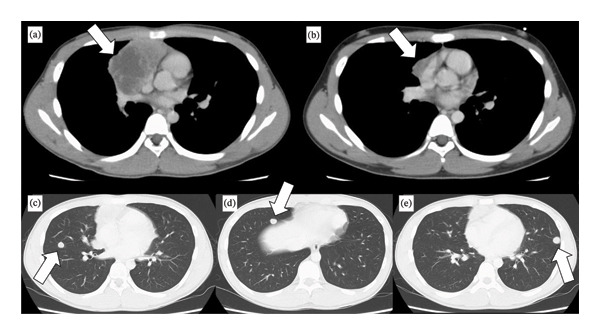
Computed tomography (CT) revealed a mass in the right anterior mediastinum and multiple bilateral lung nodules: (a) before chemotherapy, (b) at surgery after chemotherapy, and (c–e) three representative CT images of the pulmonary metastases.
